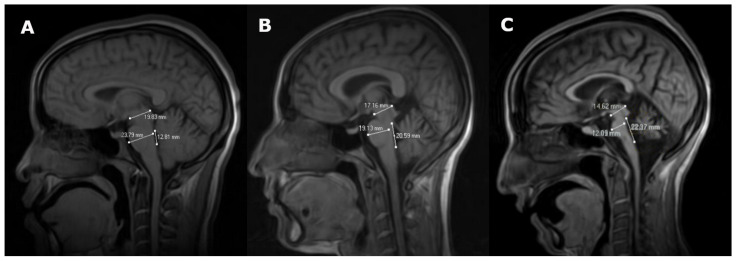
This shows how the DAPMES, DAPP, and DHIV diameters are obtained via MRI. (A) shows measurements for a control subject, (B) for a preclinical SCA2 patient, and (C) for a clinical SCA2 patient. The progression of atrophy is evident from (A–C).
